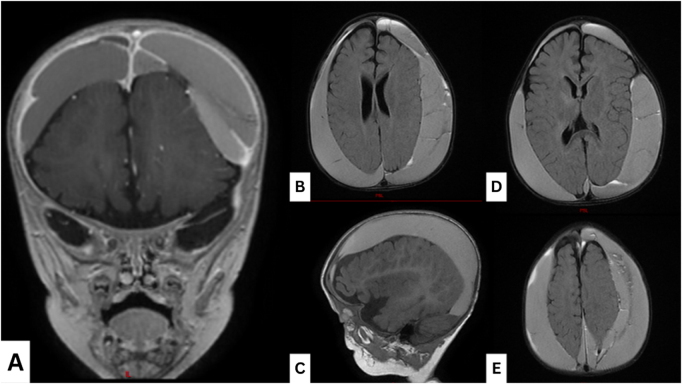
Different MRI cuts showing bilateral subacute subdural hematomas. (A) Coronal cut (top left). (B) Axial cut (top middle). (C) Axial cut (top right). (D) Sagittal cut (bottom middle). (E) Axial cut (bottom right).
